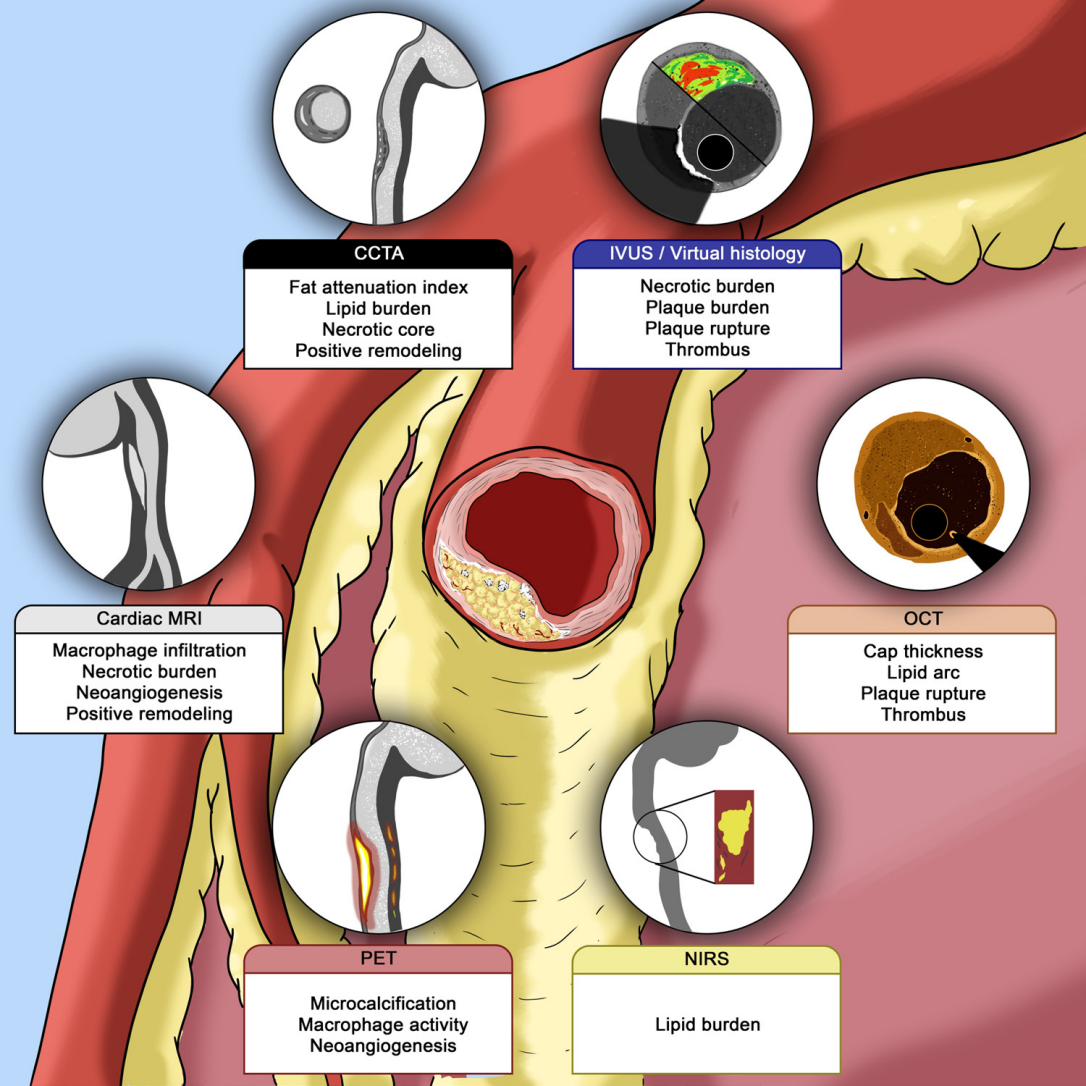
Characteristics of vulnerable plaques (VPs) by different imaging modalities. The figure summarizes the key features of VPs that can be identified by currently available invasive and noninvasive imaging techniques. A detailed discussion of each modality’s capabilities and limitations is provided in the Supplemental Material. CCTA indicates coronary computed tomography angiography; IVUS, intravascular ultrasound; MRI, magnetic resonance imaging; NIRS, near-infrared spectroscopy; OCT, optical coherence tomography; and PET, positron emission tomography.
